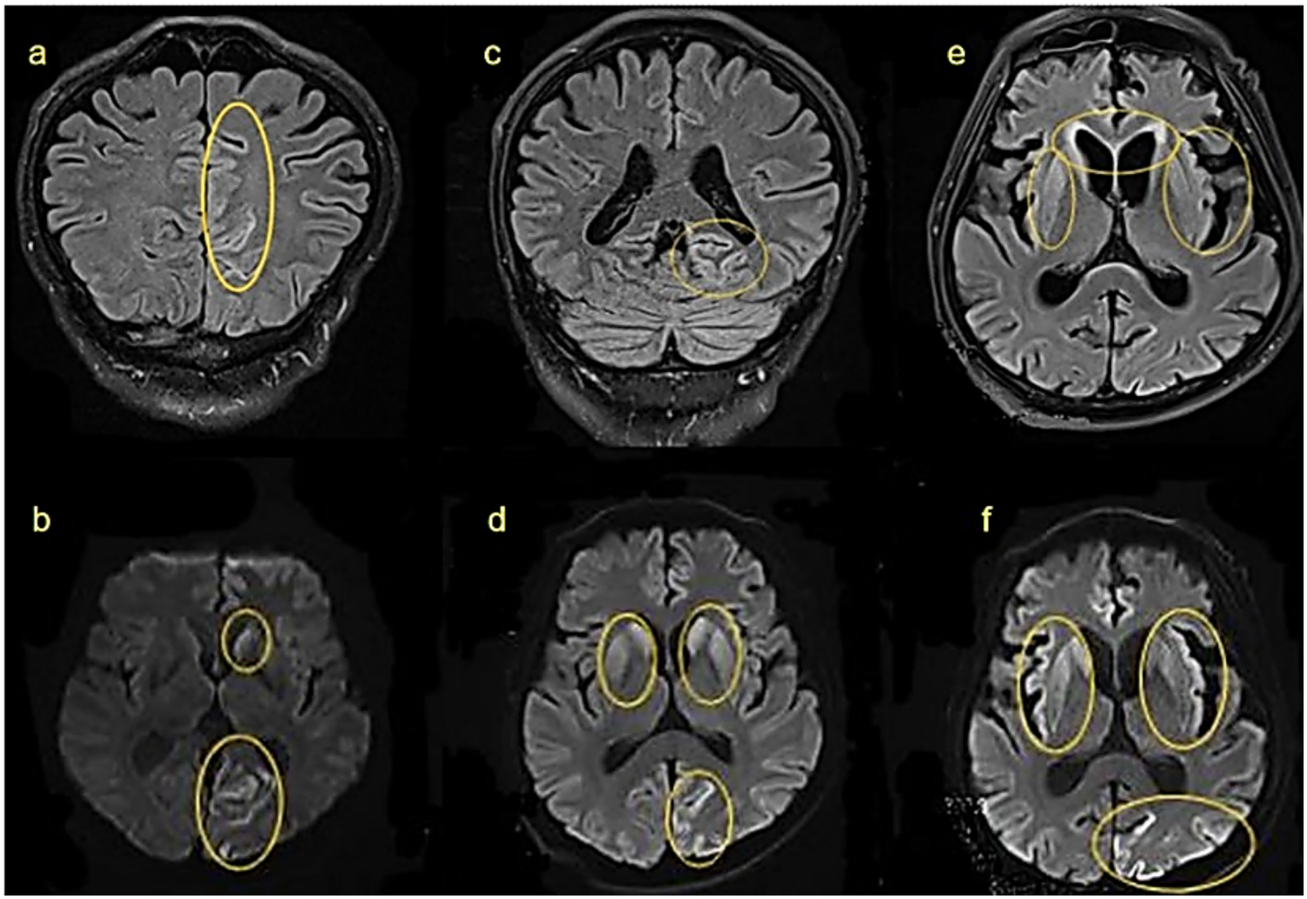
Cranial MRI findings at different stages of the same patient: (a,b) 8 weeks after onset: hyperintensities in the left caudate nucleus and left parieto-occipital cortex on FLAIR and DWI. (c,d) 12 weeks after onset: lesions extend bilaterally to caudate and lentiform nuclei. (e,f) 18 weeks after onset: further involvement of bilateral frontal lobes and insulae. Yellow circles highlight abnormal signals.
